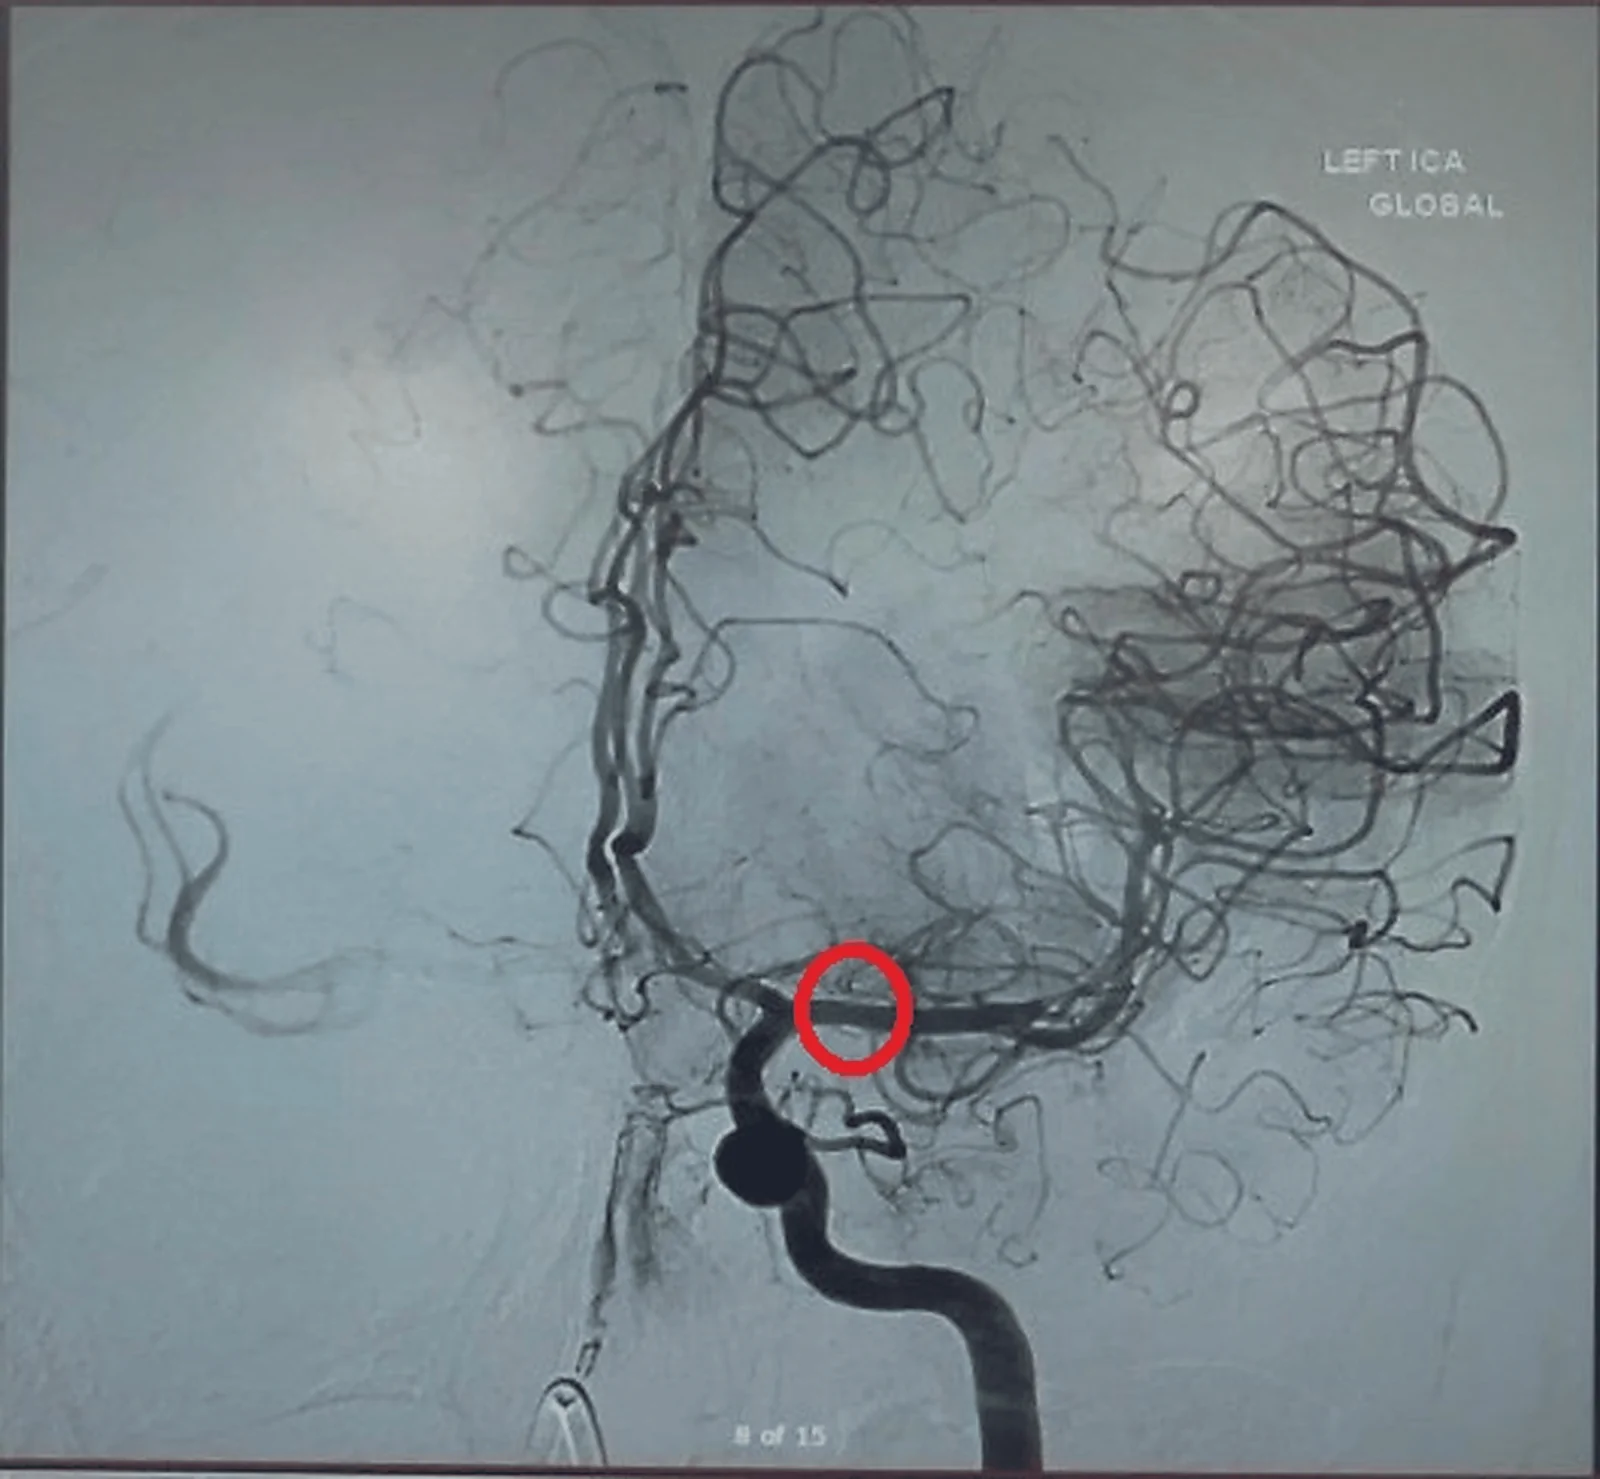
Post-thrombectomy CT angiogram Post-thrombectomy cerebral angiography demonstrating successful recanalization of the left M1 segment (circled) following repeat mechanical thrombectomy.
